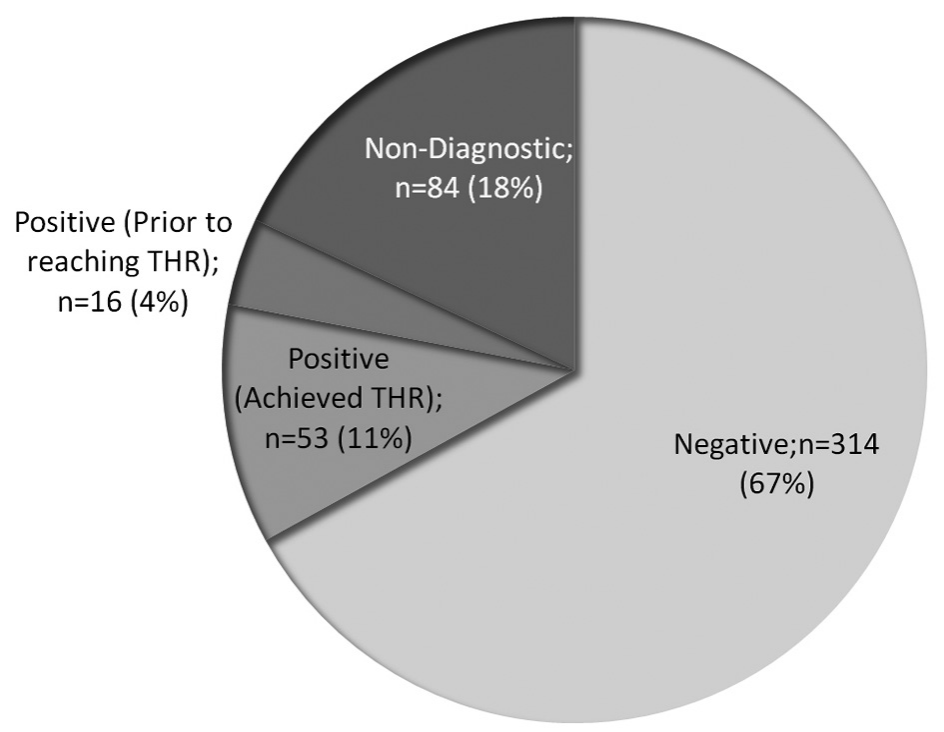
Frequency of dobutamine stress echocardiography test outcomes in patients evaluated for ischemia (n = 467). THR, target heart rate.
